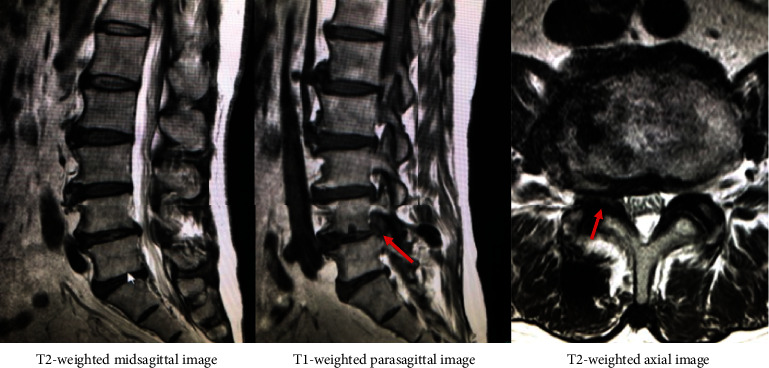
MR images revealed right L4-5 foraminal herniated disc without fragment in the spinal canal.
